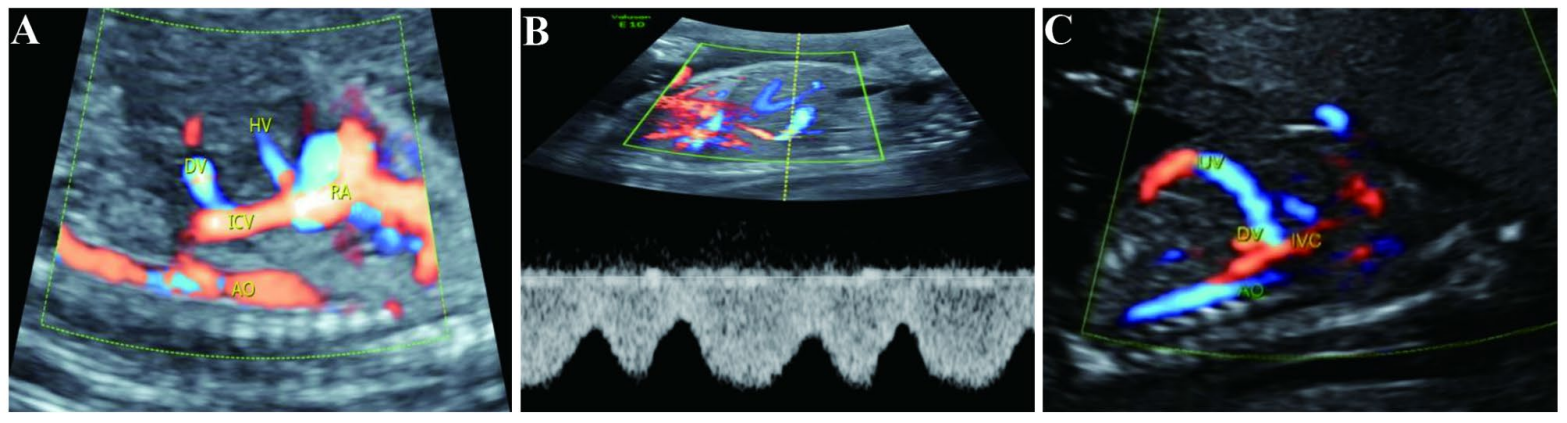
Ultrasonic images of type II UPSVS (ductus venous-systemic shunt, DVSS) (A-C) The UV-LPV-DV complexes shifted down, and the ductus venosus (DV) did not connect to subdiaphragmatic vestibule, but ectopically connected to the liver segment of inferior vena cava (IVC)
